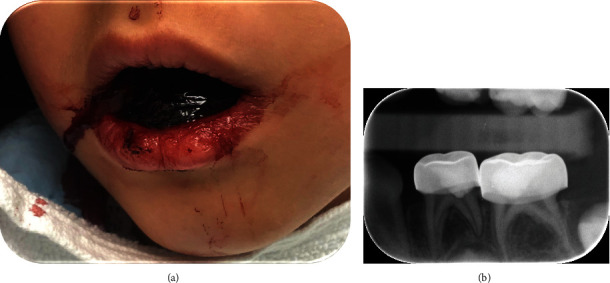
Patient case 5. (a) Excessive bleeding and a blood clot in the mouth of a girl, 16 hours after placing SSCs on her primary lower left first and second molars. (b) A periapical radiograph taken 1-day postoperatively reveals no pathology that may contribute to the excessive bleeding.
